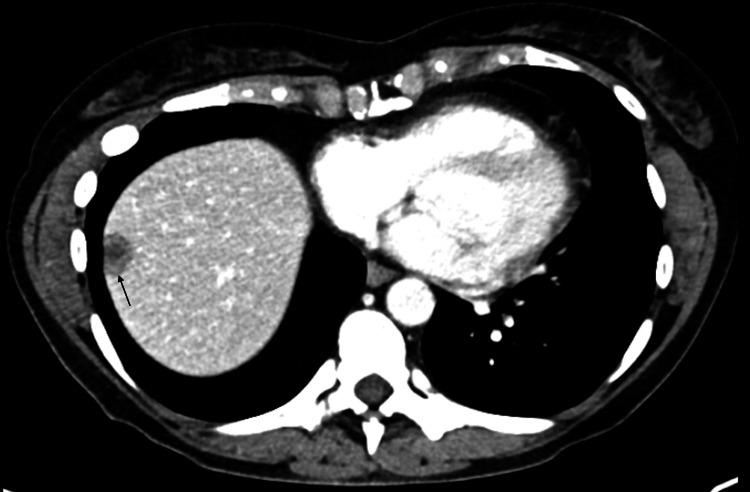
Axial CT demonstrating right hepatic lesion November 2019
